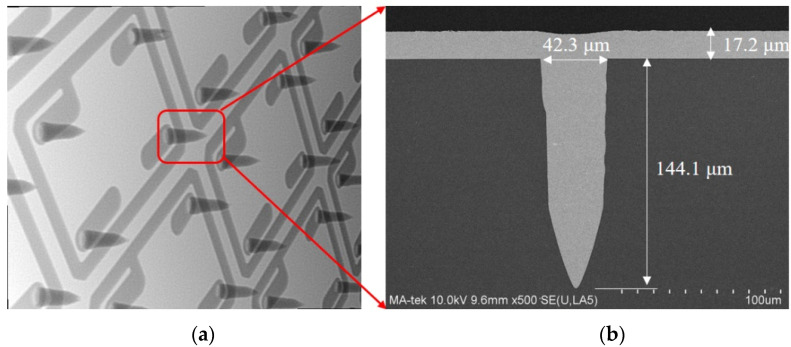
Metalized TGVs and X-ray images. (a) X-ray image. (b) Cross-sectional TGVs fully filled with Cu using bottom to up filling behavior with appropriate additive composition.
